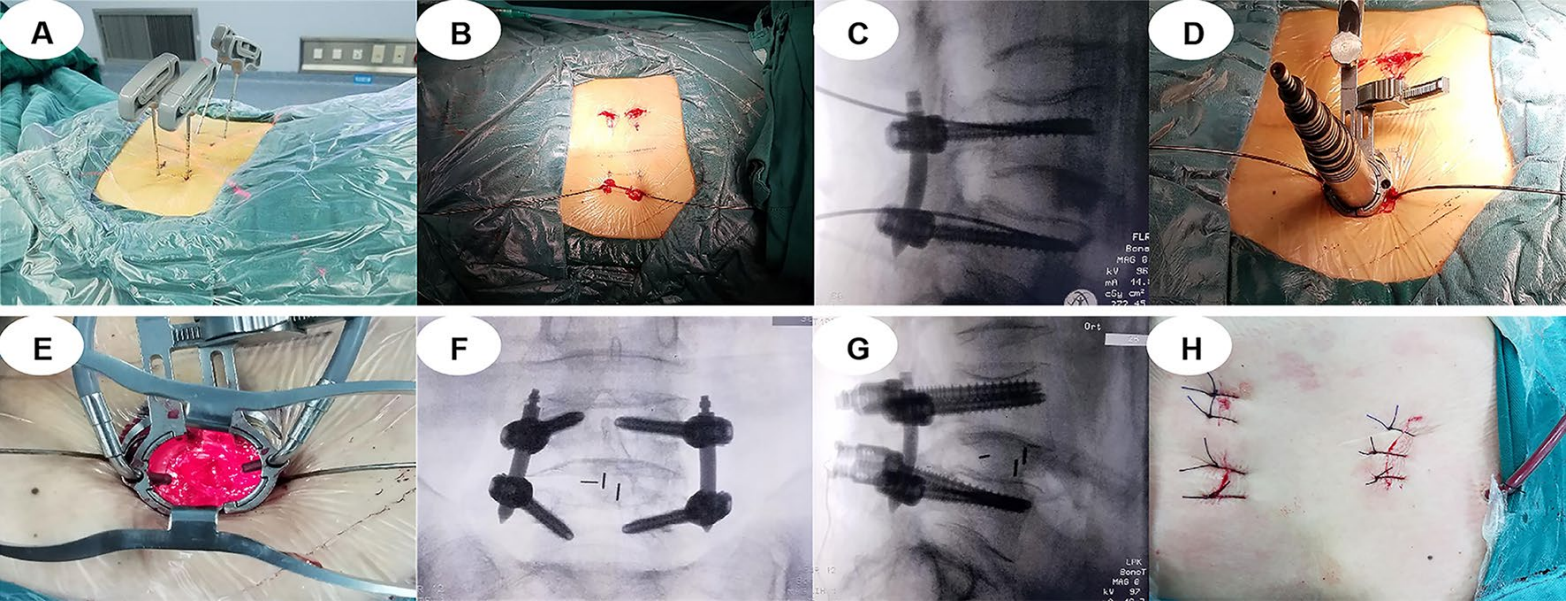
A typical MIS-TLIF case. (A–C) Posterior unilateral percutaneous pedicle screw fixation and vertebral reduction via fluoroscopy. (D) Insertion of the expansion tube and installation of the quadrant working channel. (E) Decompression and fusion under the working channel. (F–G) Position of the cage and percutaneous pedicle screws in anteroposterior and lateral X-ray views. (H) Incision and drainage tube.
